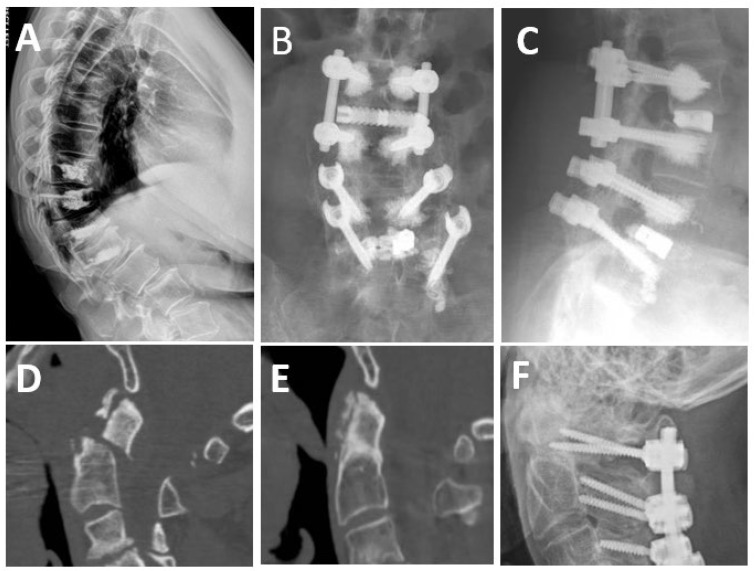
Surgical treatment options for patients with osteoporosis. (A) Four-level kyphoplasty in an elderly woman with multiple thoracic vertebral compression fractures and thoracic hyperkyphosis. (B,C) AP and lateral X-rays from a hardware revision and fusion extension in a patient with osteoporosis and adjacent segment disease (ASD) at L2/3 following solid arthrodesis from previous L3–5 fusion. Note the new screws at L2 and L3 with cement augmentation through fenestrated screws. Larger interbody cages, such as those that can be inserted from an anterolateral approach, rest on the apophyseal ring of the vertebrae and thus provide stronger anterior column support and higher fusion rates. (D) Preoperative sagittal CT scan of a patient with advanced osteoporosis and a type II traumatic odontoid fracture. (E,F) Postoperative sagittal CT and lateral X-rays of the same patient 6 months following surgical stabilization and teriparatide treatment, demonstrating reduction and healing of the fracture.
